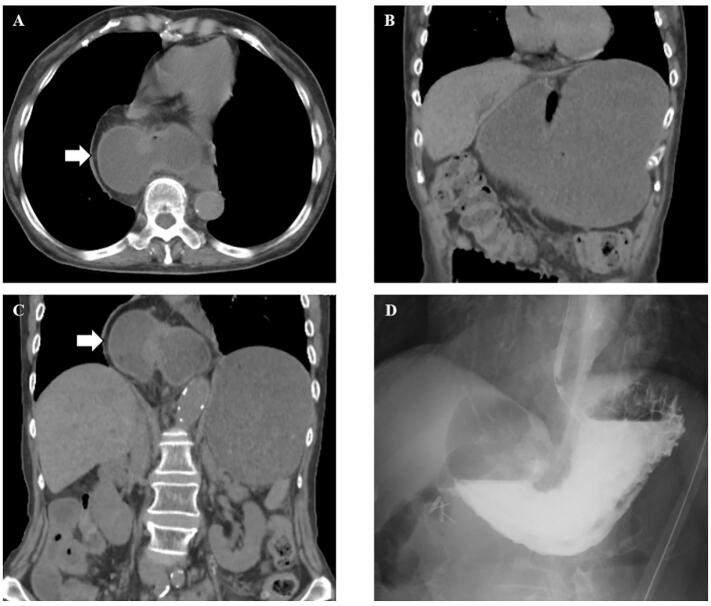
A, B, C: Duodenal prolapse and gastric dilatation within the mediastinum are observed on computed tomography (arrow indicates the duodenum bulb within the mediastinum). D: An enema shows improvement in the duodenal prolapse and gastric dilatation after decompression using a nasogastric tube.
